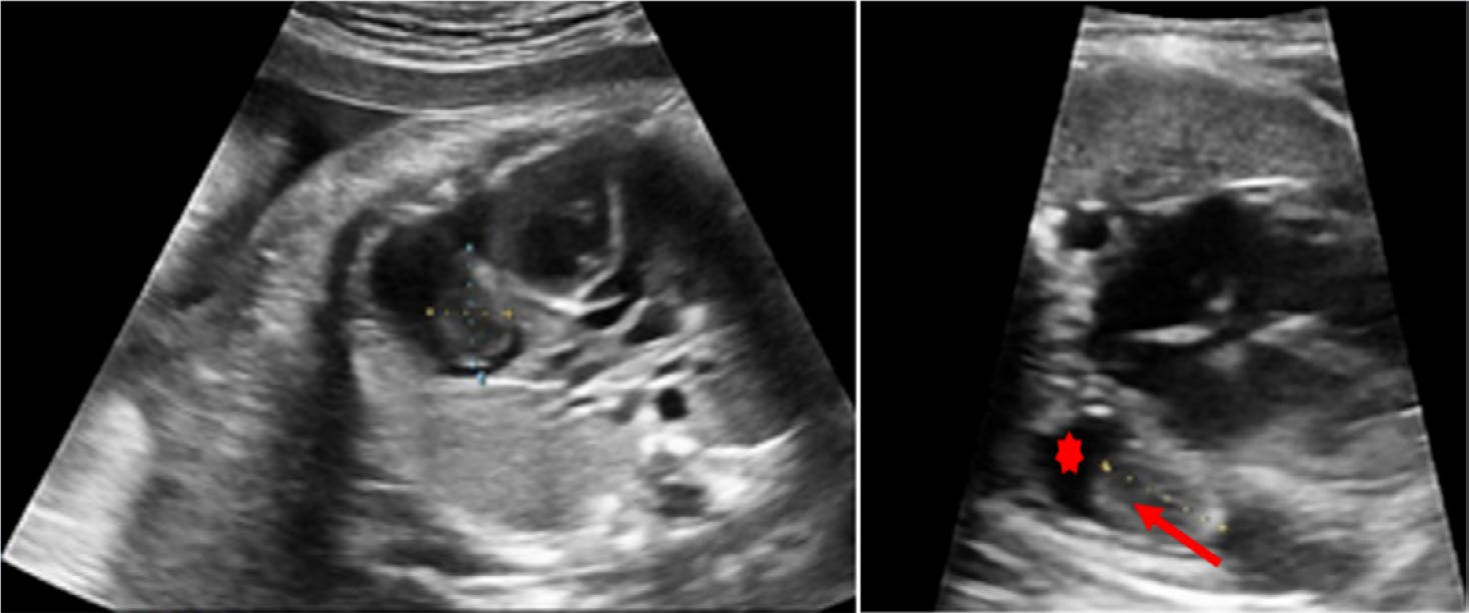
Foetal cardiac ultrasound. The non‐vascularised, heterogeneous thoracic formation skirted by (arrow) the lateral pericardial effusion (asterisk).
